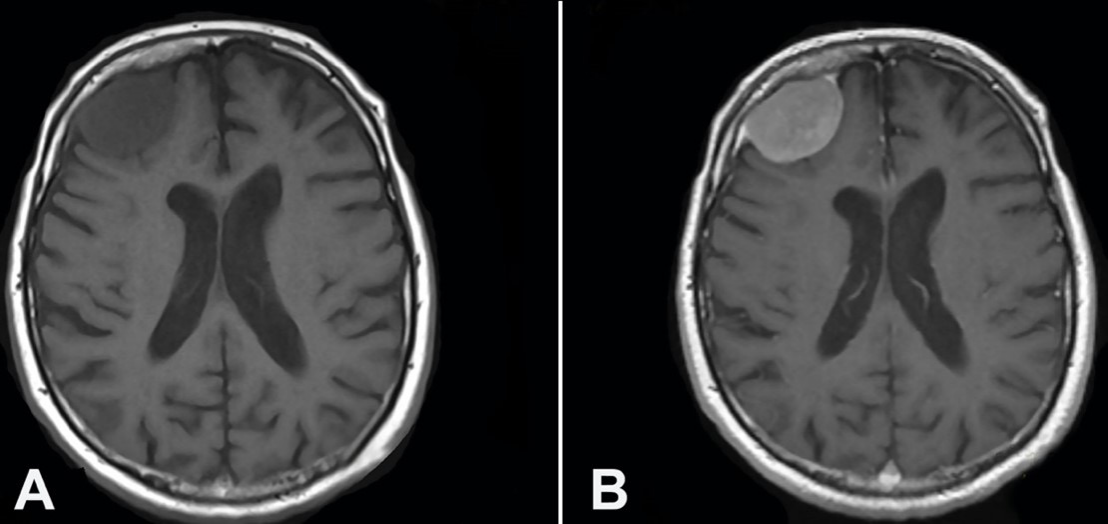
A– Preoperative Axial Brain MRI T1 weighted images showing a frontal hypointense extra-axial right lesion; B – Preoperative Axial Brain MRI T1 weighted with contrast enhancement showing homogeneous enhancement after gadolinium administration of the frontal right lesion with the characteristic dural tail.
